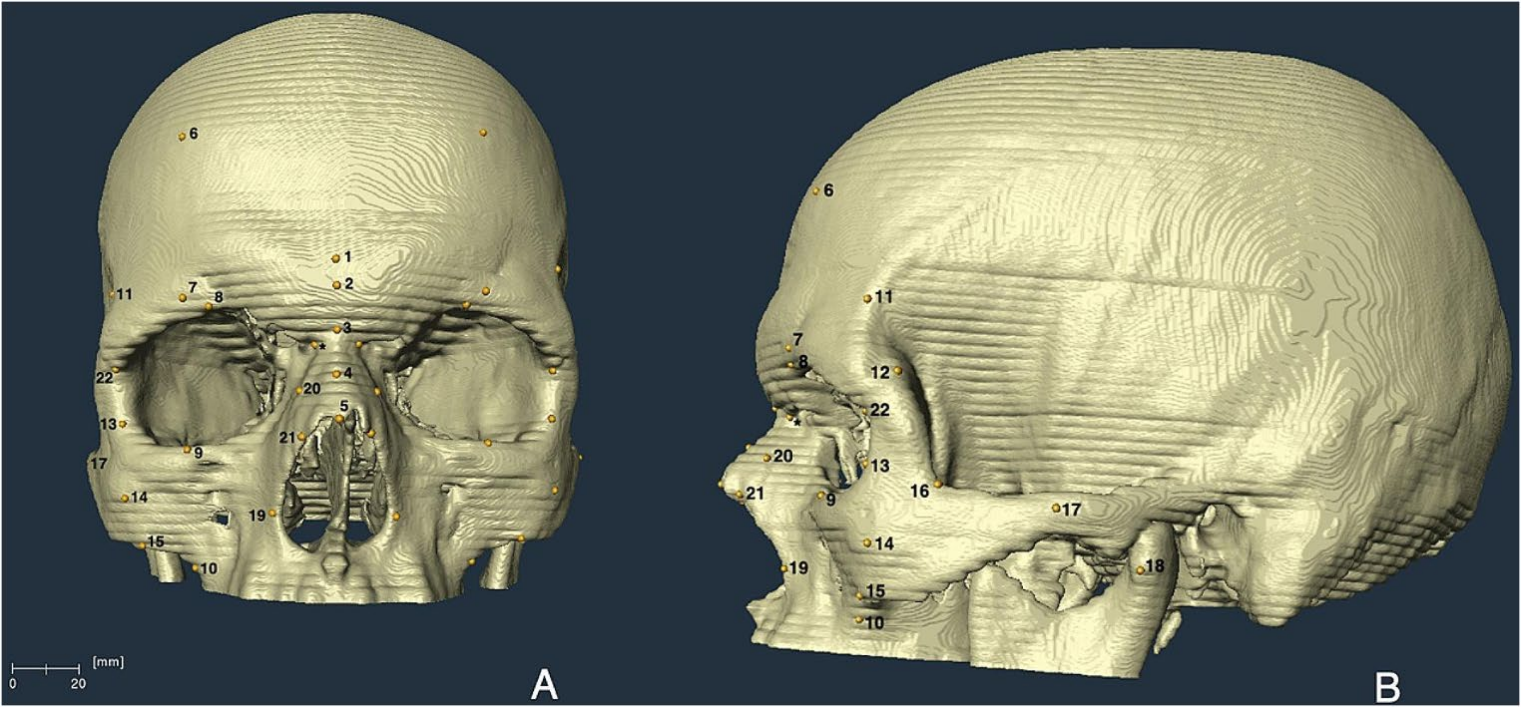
Anterior (A) and lateral (B) views of the 3D skull model, showing the cranial landmarks. (*): Nasomaxillofrontale. Due to the slice thickness of the CT scans (2.4 mm), horizontal lines appeared on the skull models
